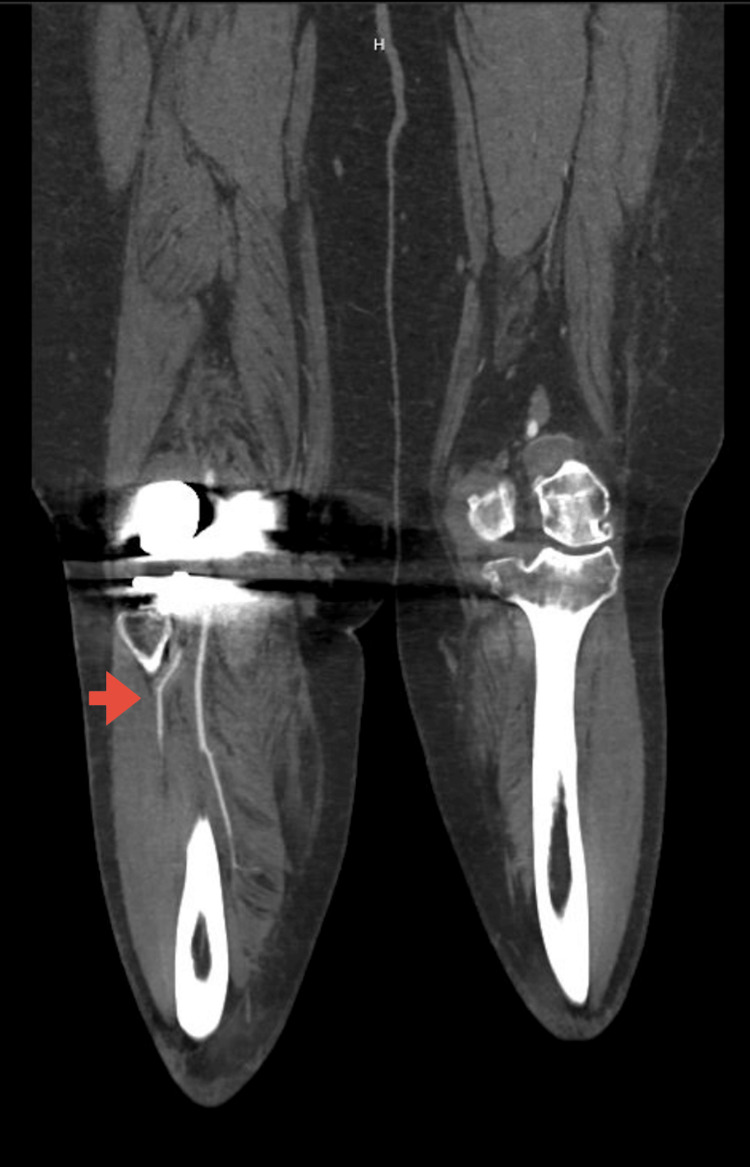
CT angiography (CTA) demonstrating contrast in the runoff vessels (red arrow), indicating the absence of vascular injury.
